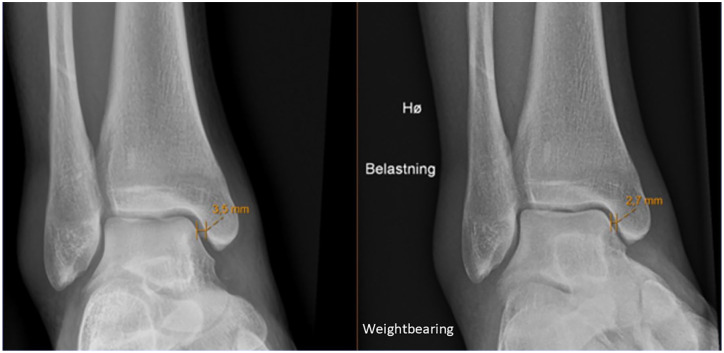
Nonweightbearing (left) and weightbearing (right) mortise view radiographs showing the difference in medial clear space.
